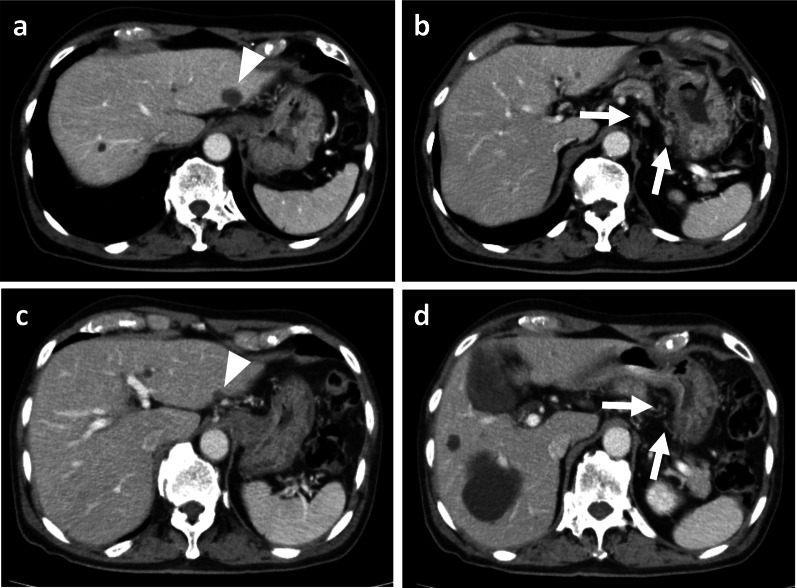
Enhanced computed tomography. a, b Before chemotherapy. Metastases at the station 3 lymph nodes (arrows) and segment 2 of the liver (arrowhead) are seen. c, d After eight courses of SOX plus T-mab. The lymph nodes (arrows) and liver metastasis (arrowhead) are remarkably reduced in size
